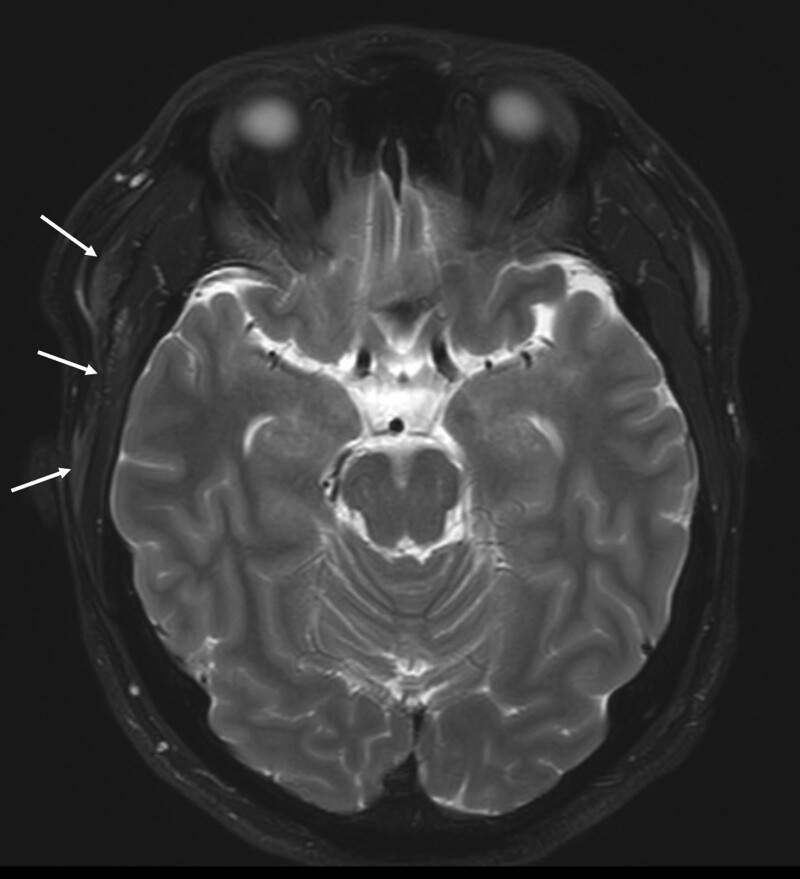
Fat-suppressed axial T2-weighted MR image reveals swelling of right temporal area and a hyperintense T2 signal lesion in the right temporal muscle, indicating myositis of the right temporal muscle. MR = magnetic resonance.
